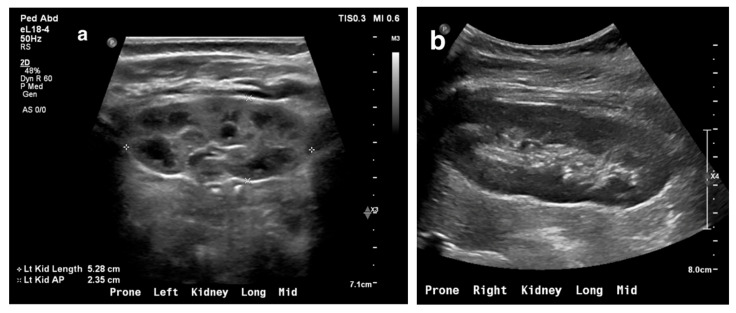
(a) Normal ultrasound of a 1-month-old infant’s kidney, showing prominent hypoechoic medullary pyramids. (b) Normal renal ultrasound of a 4-year-old child.
